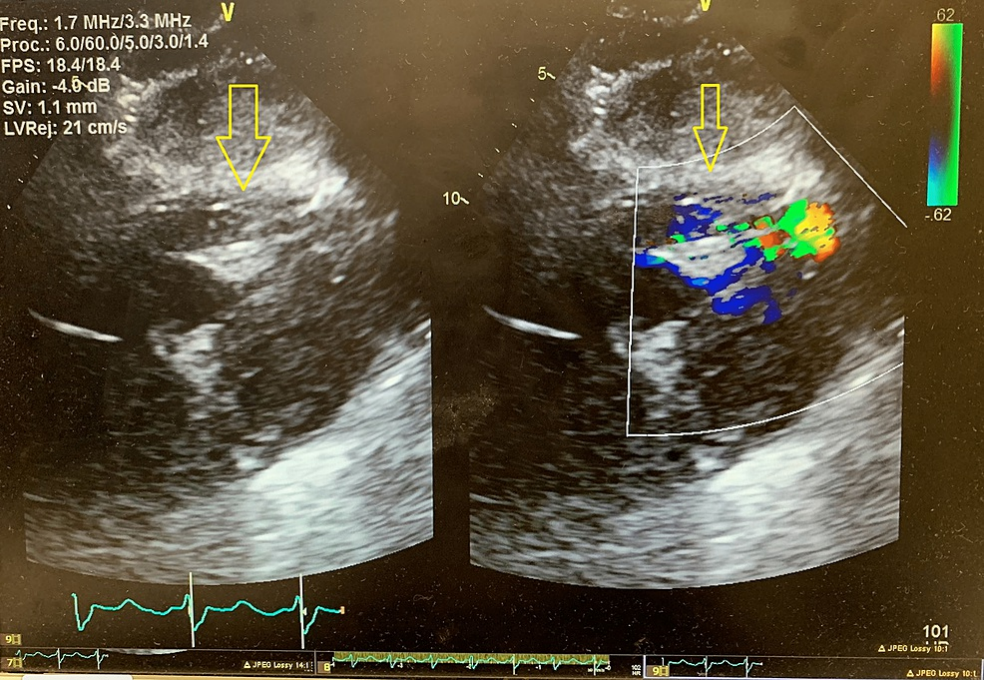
Color Doppler on transthoracic echocardiogram showing a moderately sized anteroapical ventricular septal defect with bidirectional shunting, predominantly left to right.
